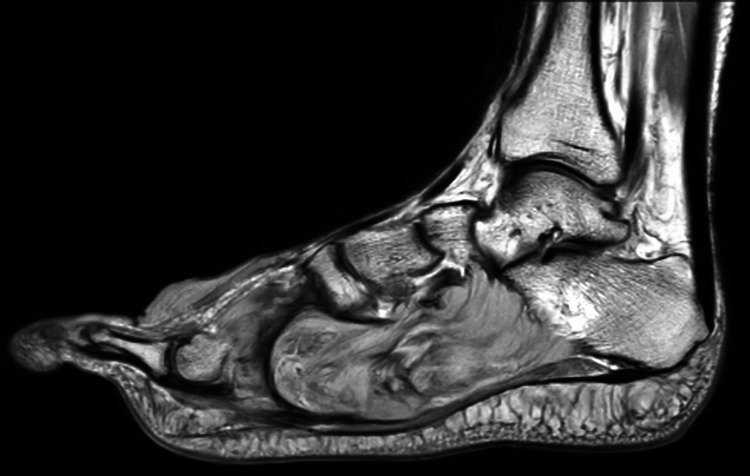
Magnetic resonance imaging (MRI) revealed an ill-defined lesion along the medial aspect of the right foot extending to the plantar region
